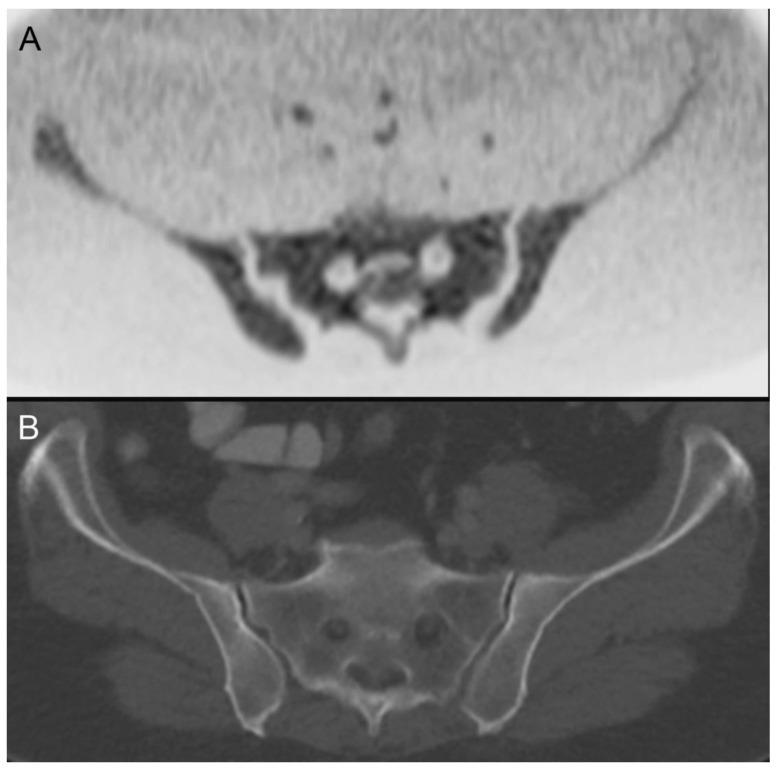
DWIBS (A) contrary to contrast-enhanced abdominal CT (B) reveals diffuse diffusion restriction of the bone marrow with no abnormalities in CT, which lead to the diagnosis of acute myeloid leukemia after further clinical work-up in this 76-year-old female patient.
